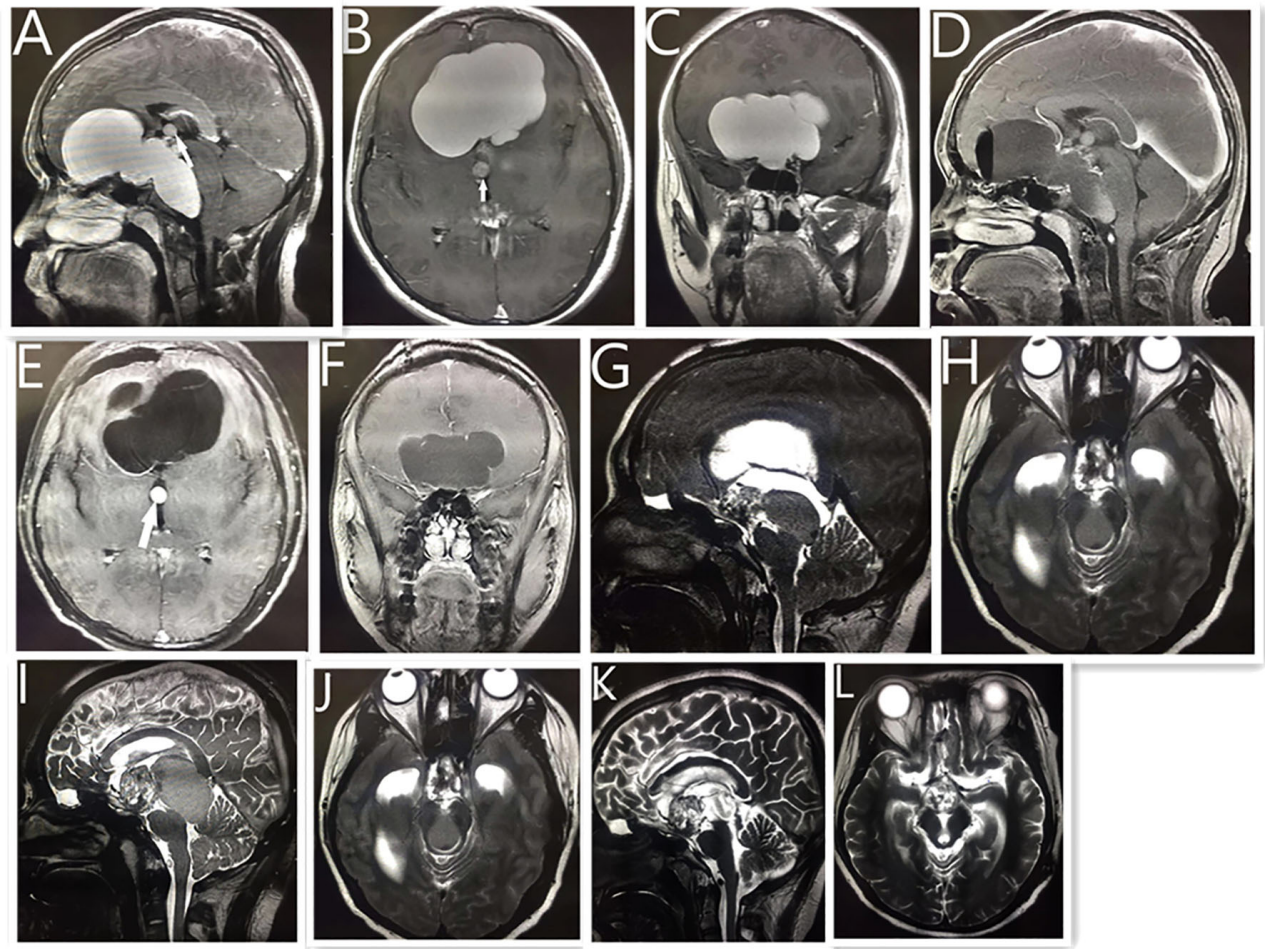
Neuroendoscopic treatment of giant cystic craniopharyngioma in a 15-year-old boy. MRI (A–C) showing a giant cystic lesion on the front middle, and posterior fossa. White arrow shows small cystic tumor of the third ventricle. Postoperative MRI reveals no obvious cystic tumor after neuroendoscopic surgery (D–F). Five years later, the MRI reveals the previous tumor had disappeared, with a solid lesion on the suprasellar region (G) and a cystic lesion compressing the mid brain aqueduct (H), which caused hydrocephalus. The patient received a ventriculoperitoneal shunt, and his condition improved. One year later, MRI reveals (I, J) that the tumor had become larger than before. Postoperative MRI reveals that (K, L) the cystic lesion disappeared and the midbrain aqueduct opened up after the neuroendoscopic surgery. As of 2 years into follow-up, the size of the remaining tumor had not changed, and the patient had continued to live a normal life.
